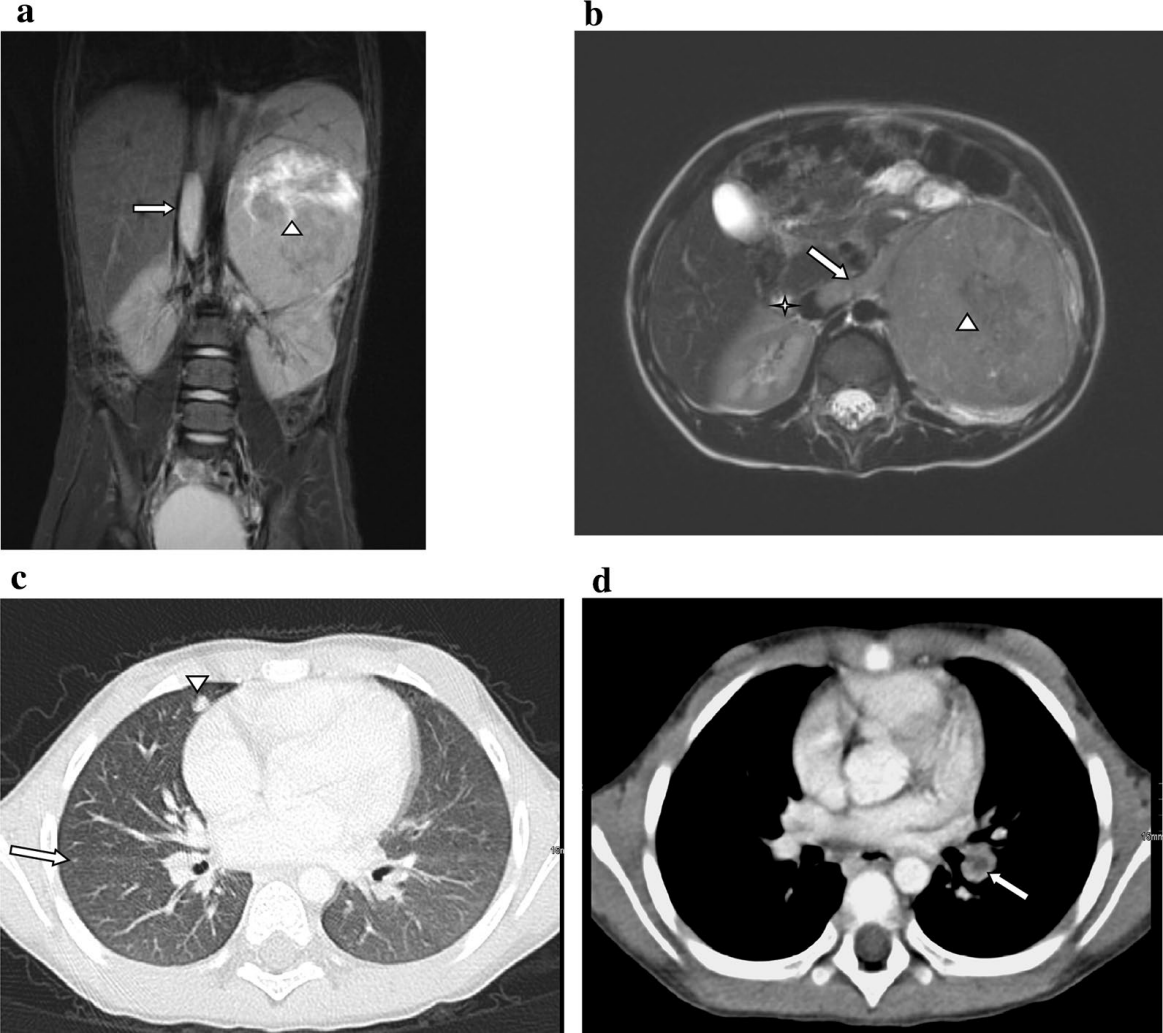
MRI abdomen at initial diagnosis (T2-weighted images). a (Coronal). Left-sided abdominal mass (*triangle*) arising from the upper pole of the left kidney and tumor thrombus in the vena cava inferior (*arrow*). b (Transversal). Main tumor formation (*triangle*) and tumor cone in the left vena renalis (*arrow*) extending to the vena cava inferior (*asterisk*). Computed tomography scan of the lungs. c The *arrows* indicate the presence of lung metastases in the middle lobe (*reverse triangle*) and right inferior lobe close to the pulmonary fissure (*arrow*). d Embolic occlusion (*arrow*) of the left inferior lobe artery by either a thrombus or tumor
